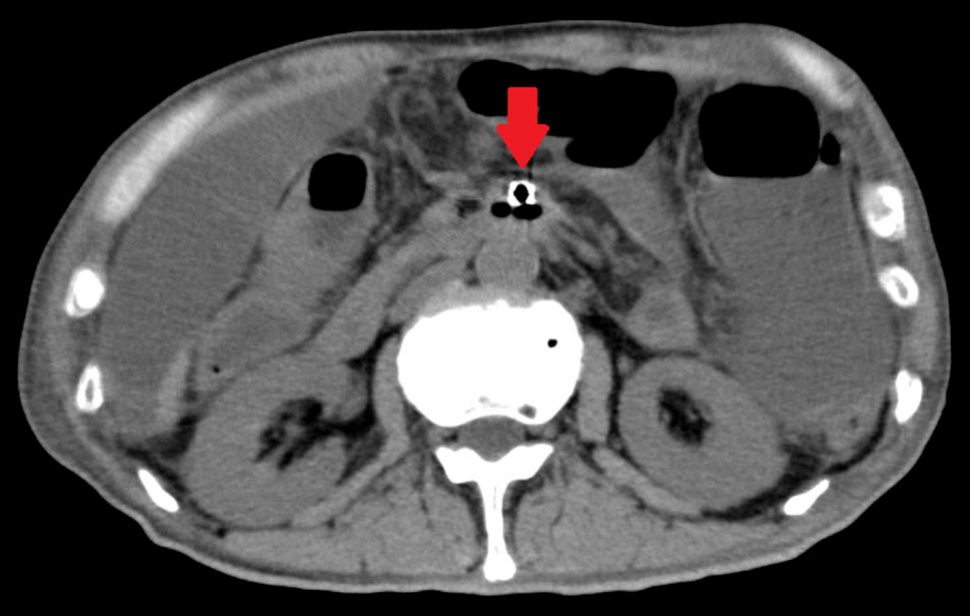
Computed tomography showed an air embolism of the SMA (*down arrow*), massive abdominal fluid collection and extensive mesenteric ischemia
